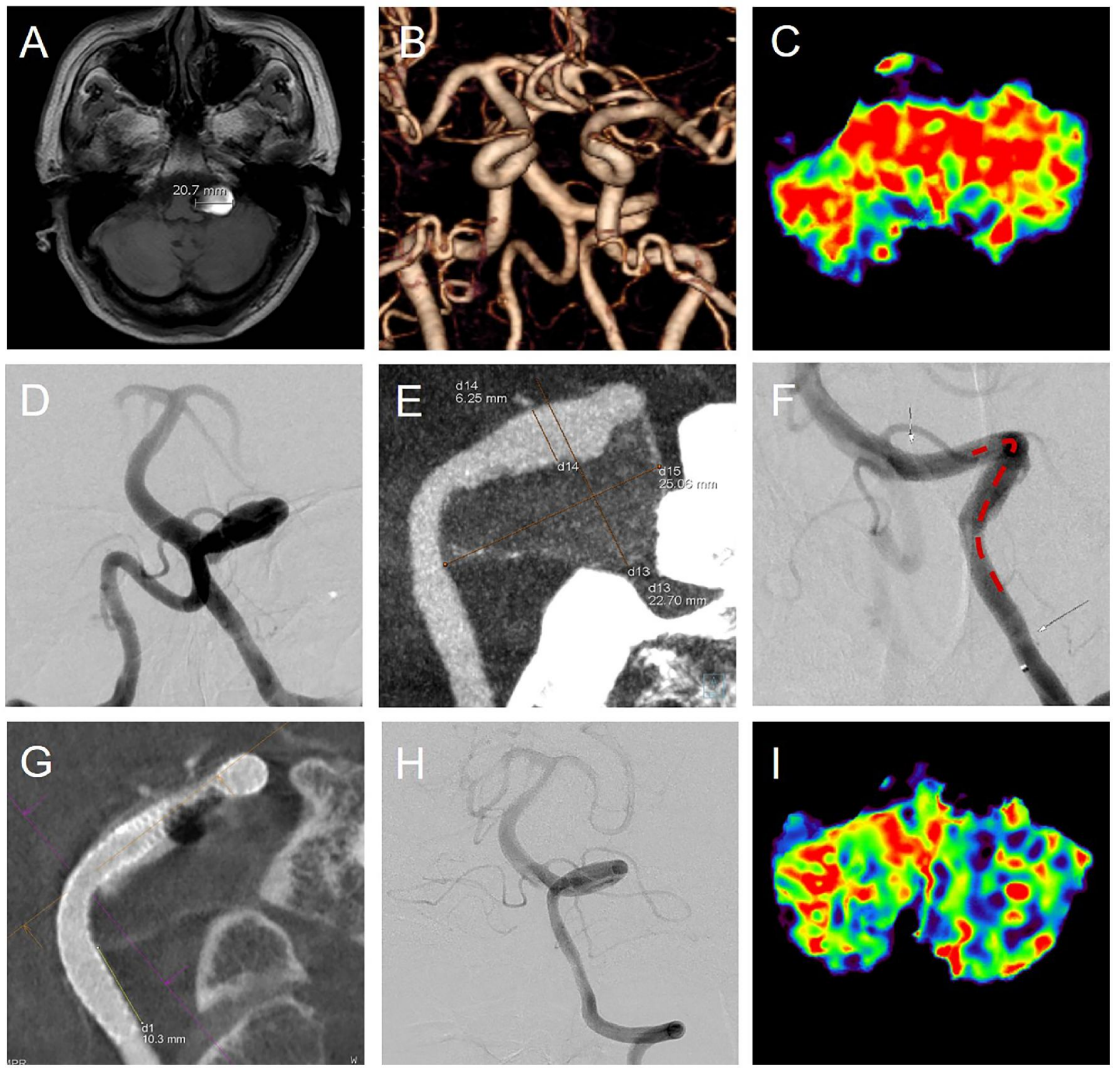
Preoperative assessment, treatment, and 8-month follow-up of a CTA-negative giant VADA. (A) Shows a mixed signal in the CPA region (max diameter: 20.7 mm). CTA (B) reveals normal V4 segment diameter, but (C) shows posterior circulation hypoperfusion. DSA (D) identifies left V4 tortuosity without abnormal dilation. DCE-CT (E) detects a giant left VADA (25.06 mm × 22.70 mm). A flow diverter (Surpass Evolve, 5 mm/40 mm) was implanted, extending 10 mm beyond the lesion margins (F; white arrows mark stent ends, red dashed line indicates lesion length). (G) confirms the stent extends 10.3 mm beyond the VADA edge. After 8 months (H), facial numbness and dysarthria resolved, and posterior circulation perfusion normalized (I).
